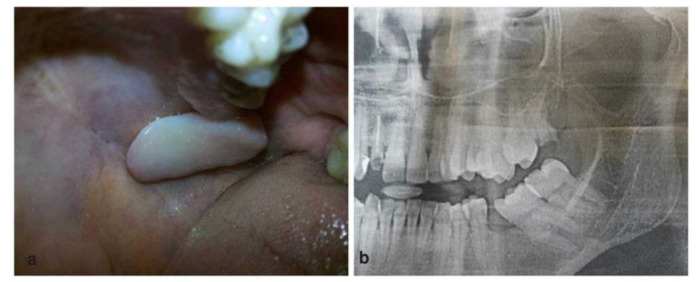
a: Clinical picture of the lesion presented on the posterior palate, b: Panoramic radiograph reveals that lesion was not associated with the underlying bone
